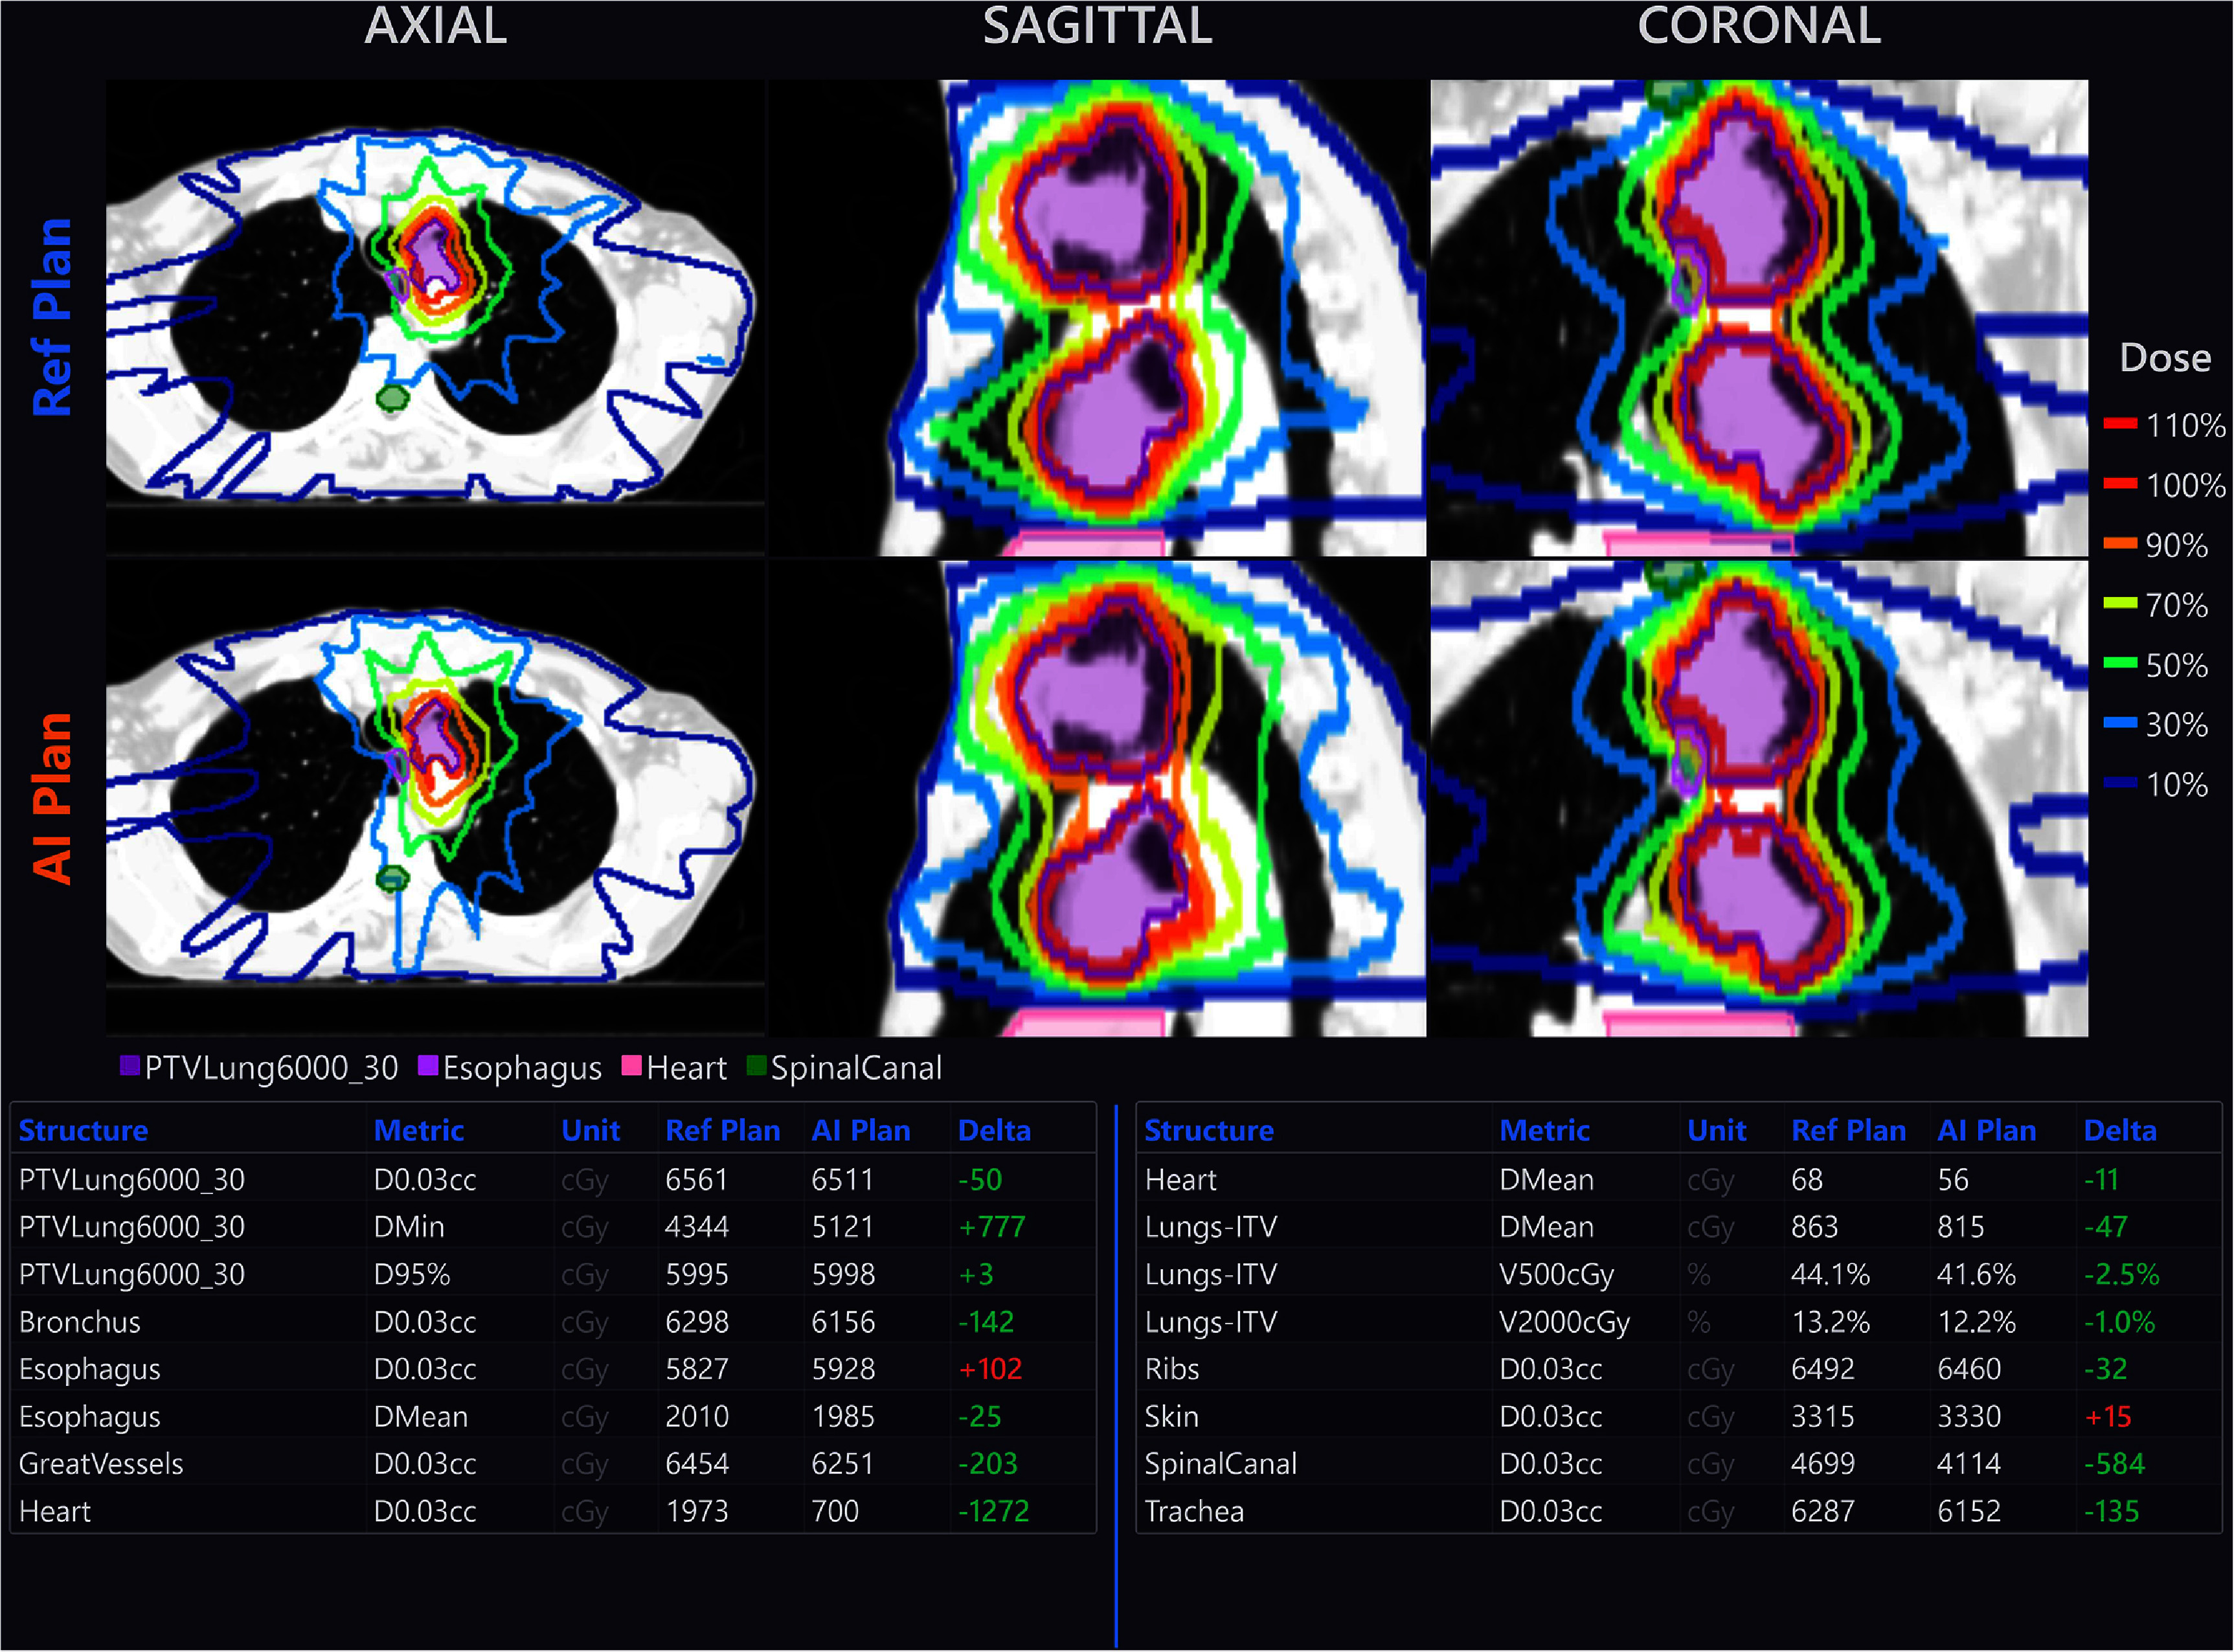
A lung case that receives 60 Gy in 30 fractions. Top row: clinical reference plan. Bottom row: artificial intelligence (AI) plan. Dose is overlaid on axial computed tomography (CT) images with the planning target volume (PTV) (purple), esophagus (light purple), heart (pink), and spinal canal (green) contours displayed. Below the images is a dose-volume histogram (DVH) metric comparison between the two plans. The AI plan outperformed the clinical reference plan in most metrics, most particularly in the minimum dose delivered to the PTV.
